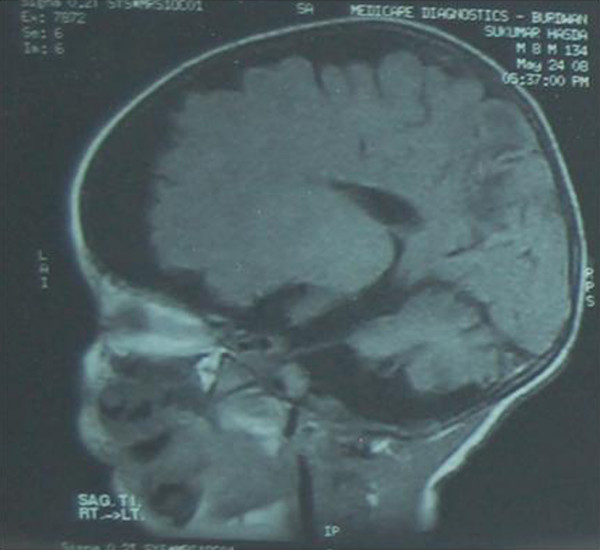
The MRI study of the brain revealed prominent bilateral extra-axial C.S.F. spaces with gliosis in both posterior parieto-occipital area with prominent left lateral ventricle and cerebellar folias.
